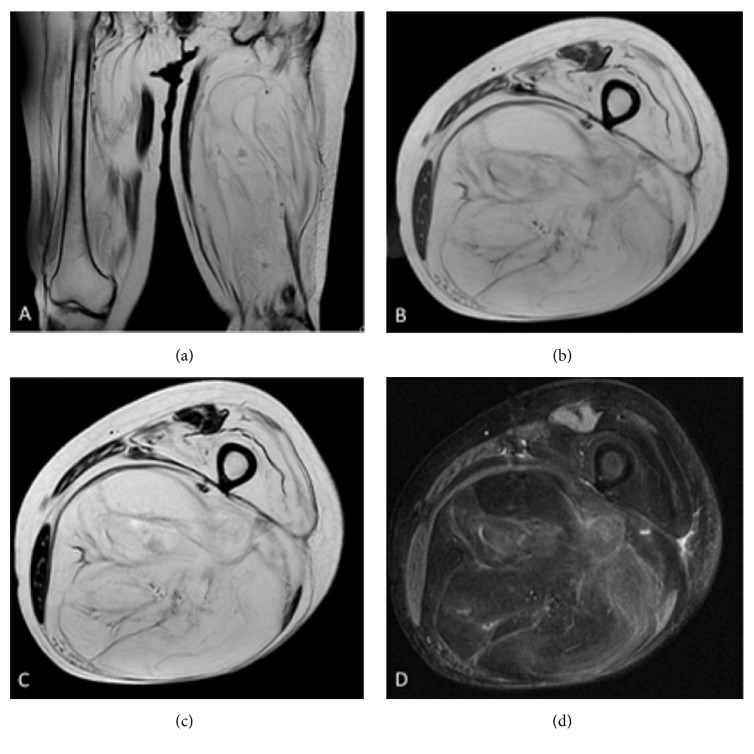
Magnetic resonance imaging revealed a tumor mass measuring 13 × 15 × 24 cm located in the left posterior thigh. Almost all the muscles, except the rectus femoris, sartorius, and gracilis muscles, show lipomatous changes. T1- and T2-weighted images (WIs) T2-WI, and short-TI inversion recovery (STIR) images show high intensity. Coronal T2-WI (a), T1-WI (axial view) (b), T2-WI (axial view) (c), and STIR images (axial view) (d).
